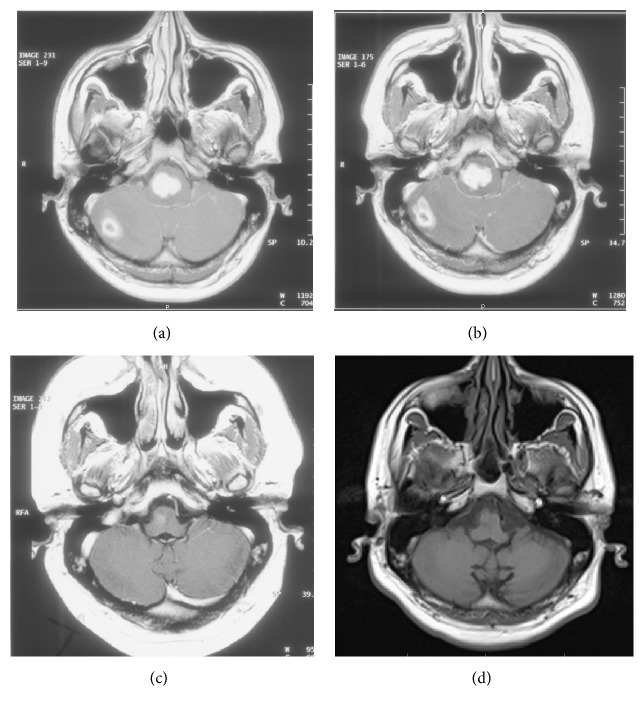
MRI scan during treatment phases. At diagnosis (a), one month after diagnosis on HREZ (b), after two months of therapy based on resistance assay (c), and after five years (d).
